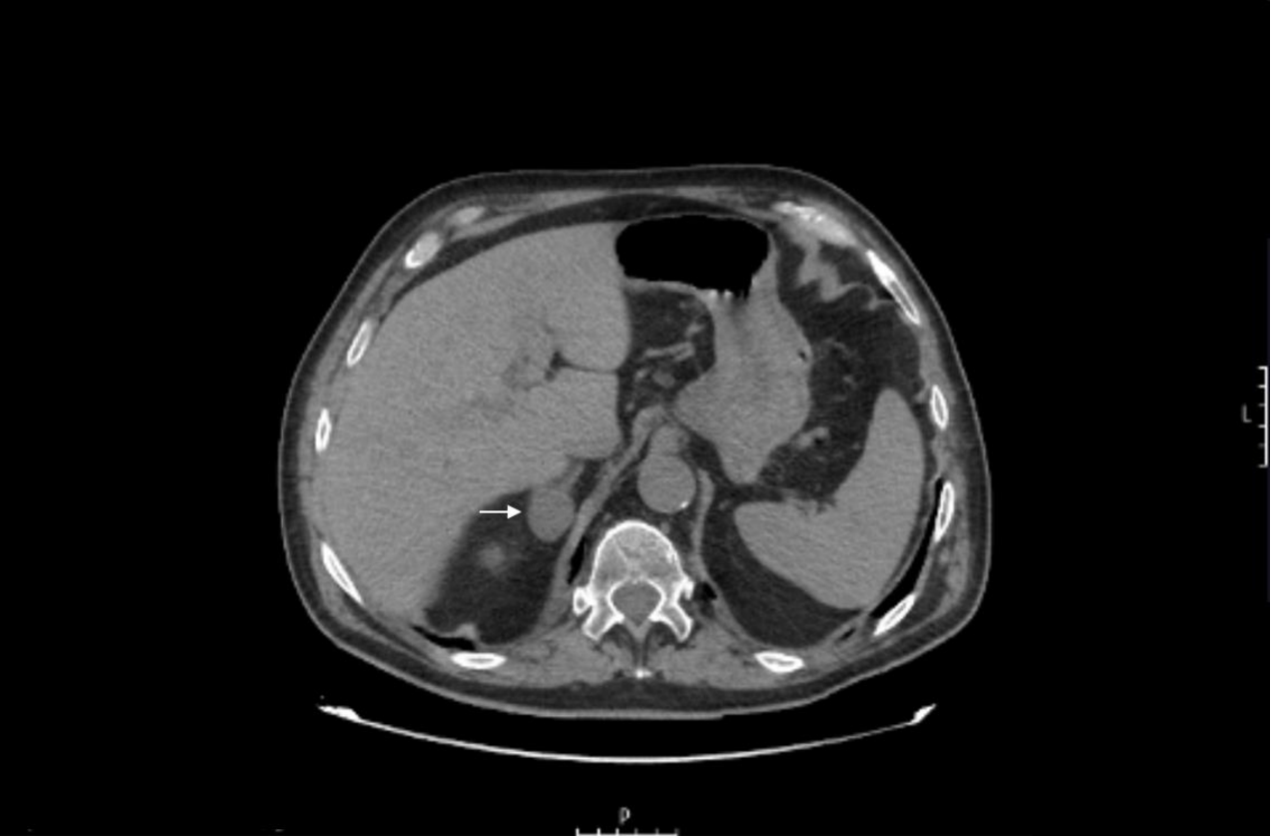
Adrenal computed tomography of the case study. White arrow showing a 27-mm nodule in the right adrenal gland.
